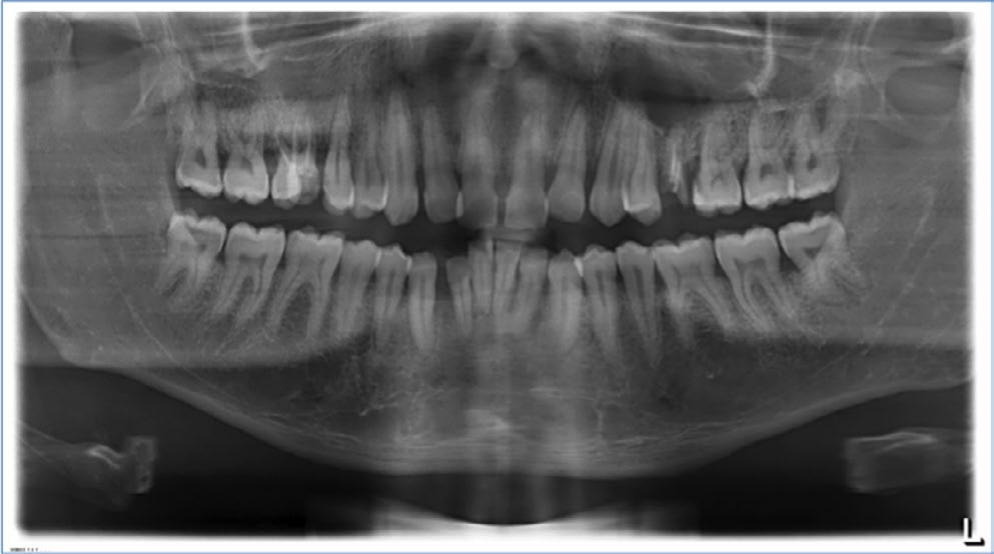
Orthopantomogram of the patient with diverse carious lesions with conservative treatment needs, and root remnants 16 and 25 with radicular cysts
